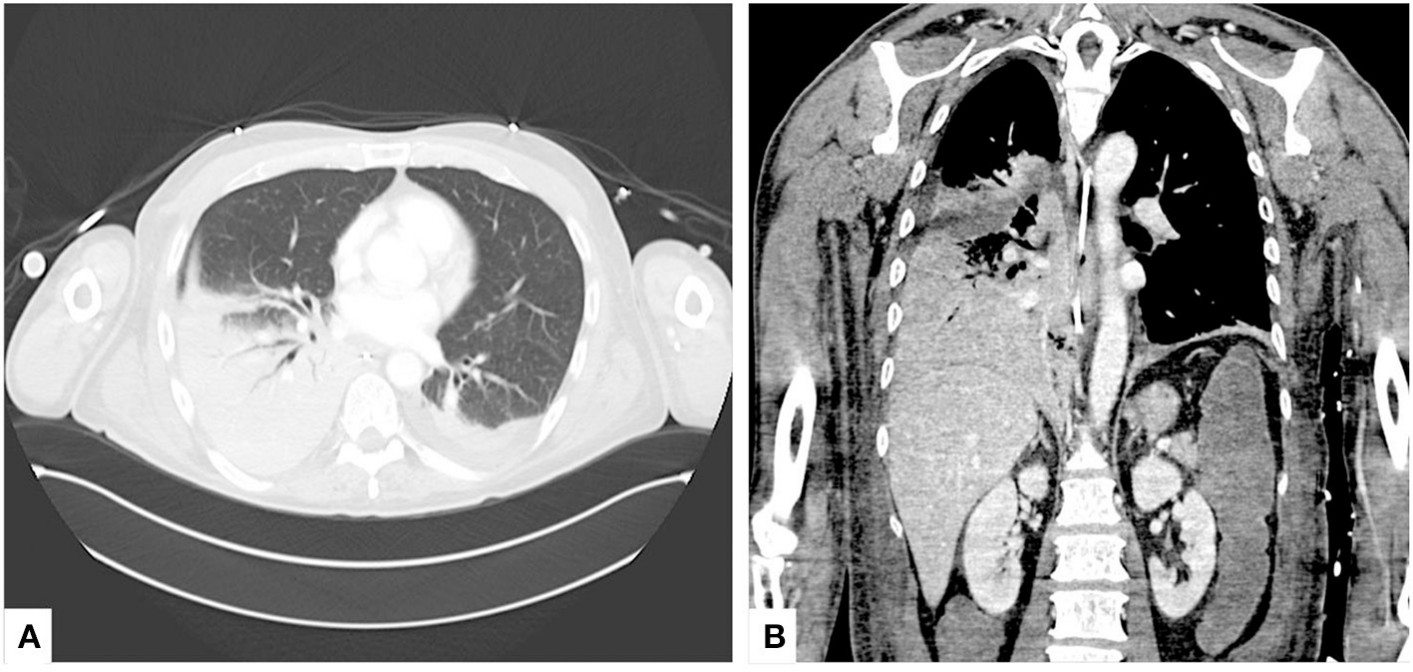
Preoperative CT scan. (A) Axial and (B) coronal views of admission chest CT scans showing mixed ground-glass opacities and consolidation with positive air bronchograms involving both the medium and the lower right lobes.
